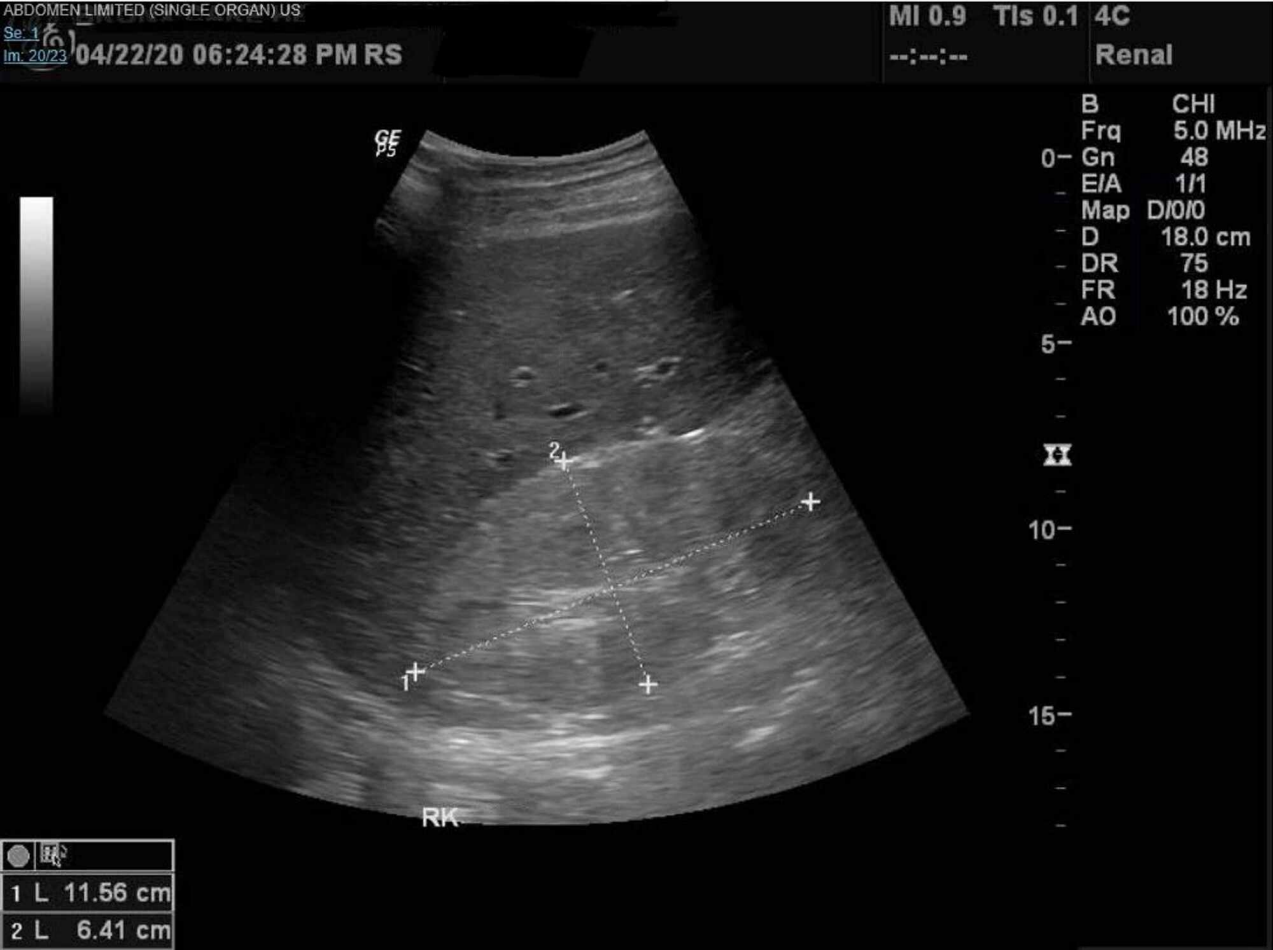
Renal Ultrasound - Right kidney
